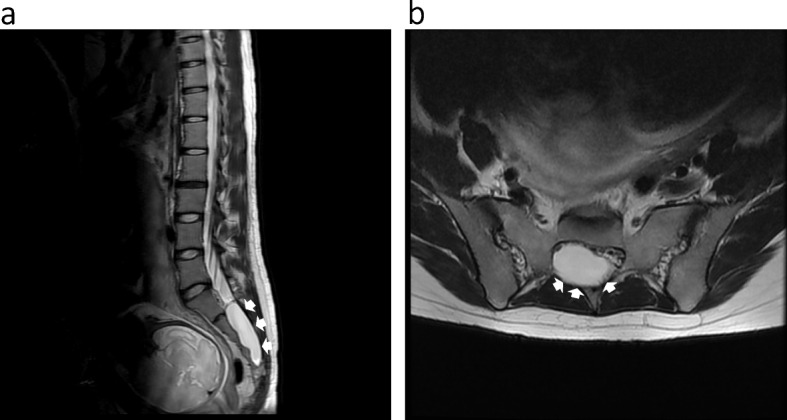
Magnetic resonance imaging for a pregnant woman with tethered cord syndrome and Tarlov cyst. a Sagittal T2-weighted image demonstrates fixation (tethering) of filum terminale on the anterior spinal canal, and the conus medullaris ended at L4. b Coronal image demonstrates the Tarlov cyst (sacral cyst in the spinal canal, arrows)
